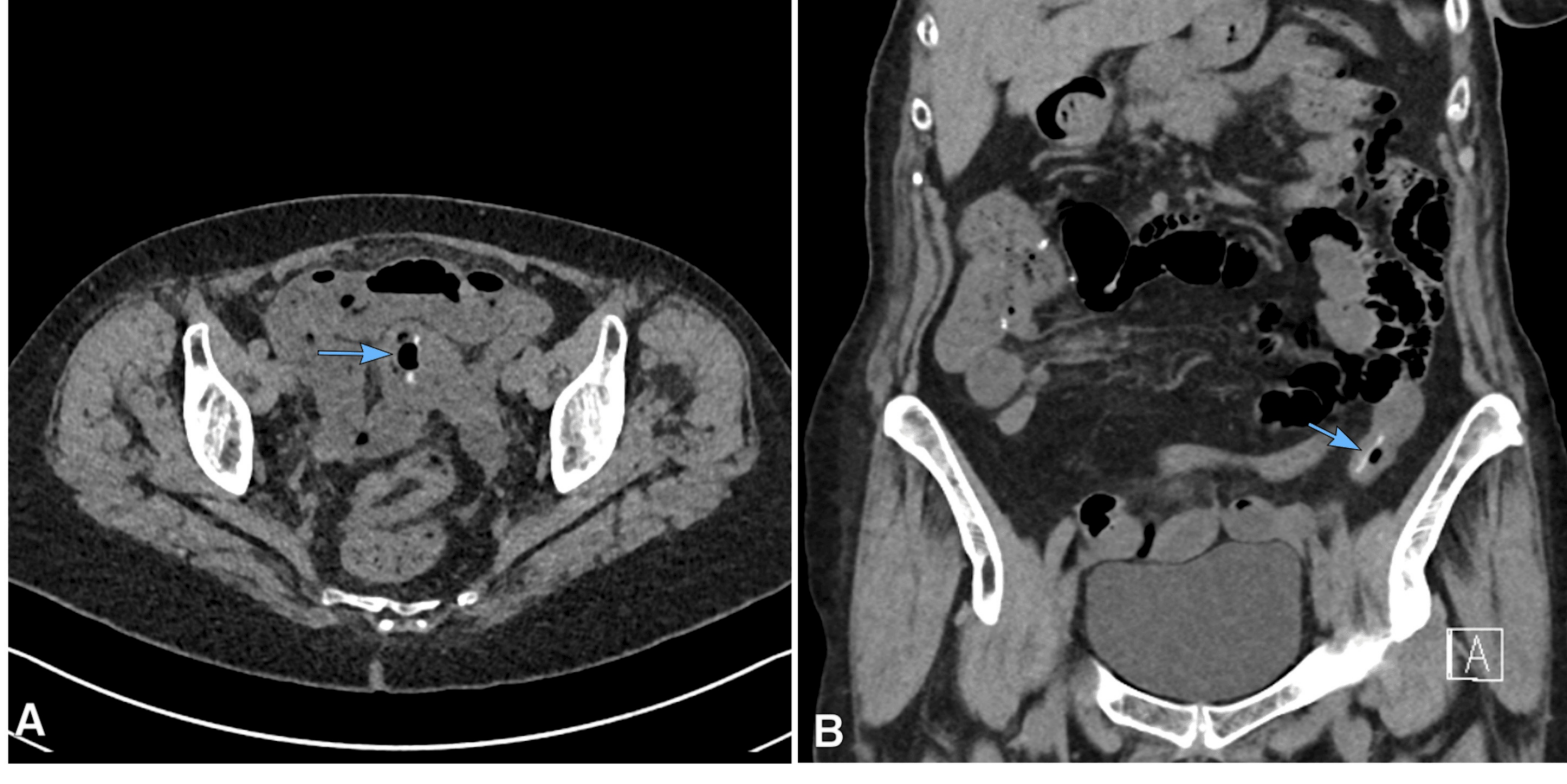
Non-enhanced abdominal CT scan at the time of initial presentation A spontaneously hyperdense structure (blue arrow) is seen within the ileum in the lower left quadrant. Axial view (A); coronal view (B).
